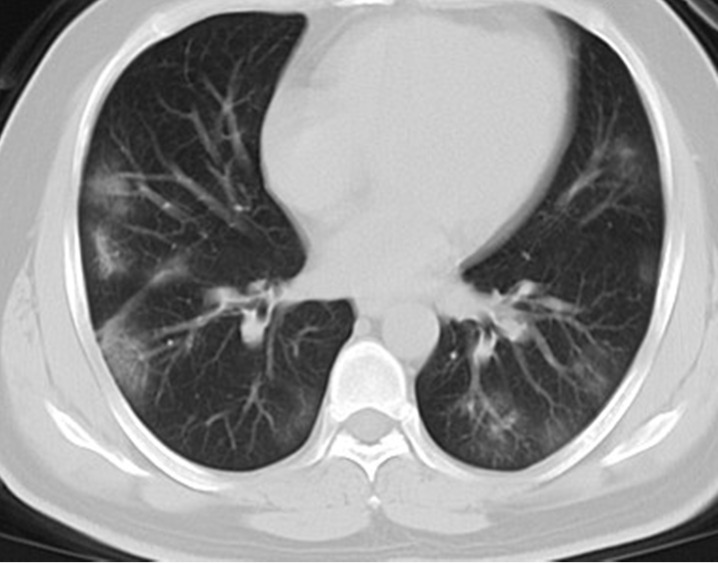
Initial chest CT of a 41-year-old man who presented with fever and cough for 3 days. Bilateral multiple patchy ground glass opacities (GGOs), with peripheral distribution were seen. The patient was discharged 6 days later after symptom improvement.
